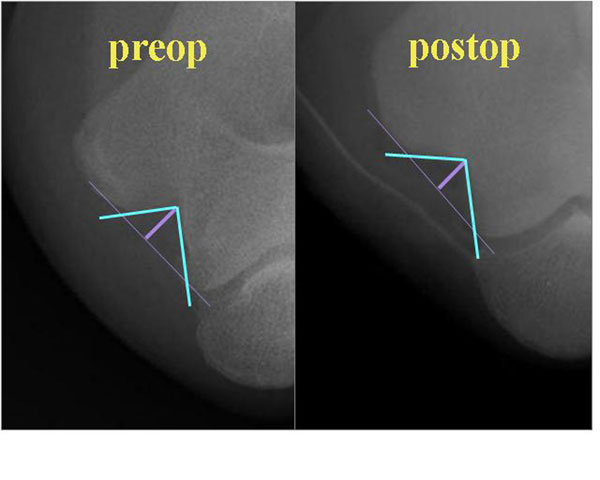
Both cubital tunnel angle (CTA) and cubital tunnel depth (CTD) became wider and shallower after minimal medial epicondylectomy and in situ decompression
